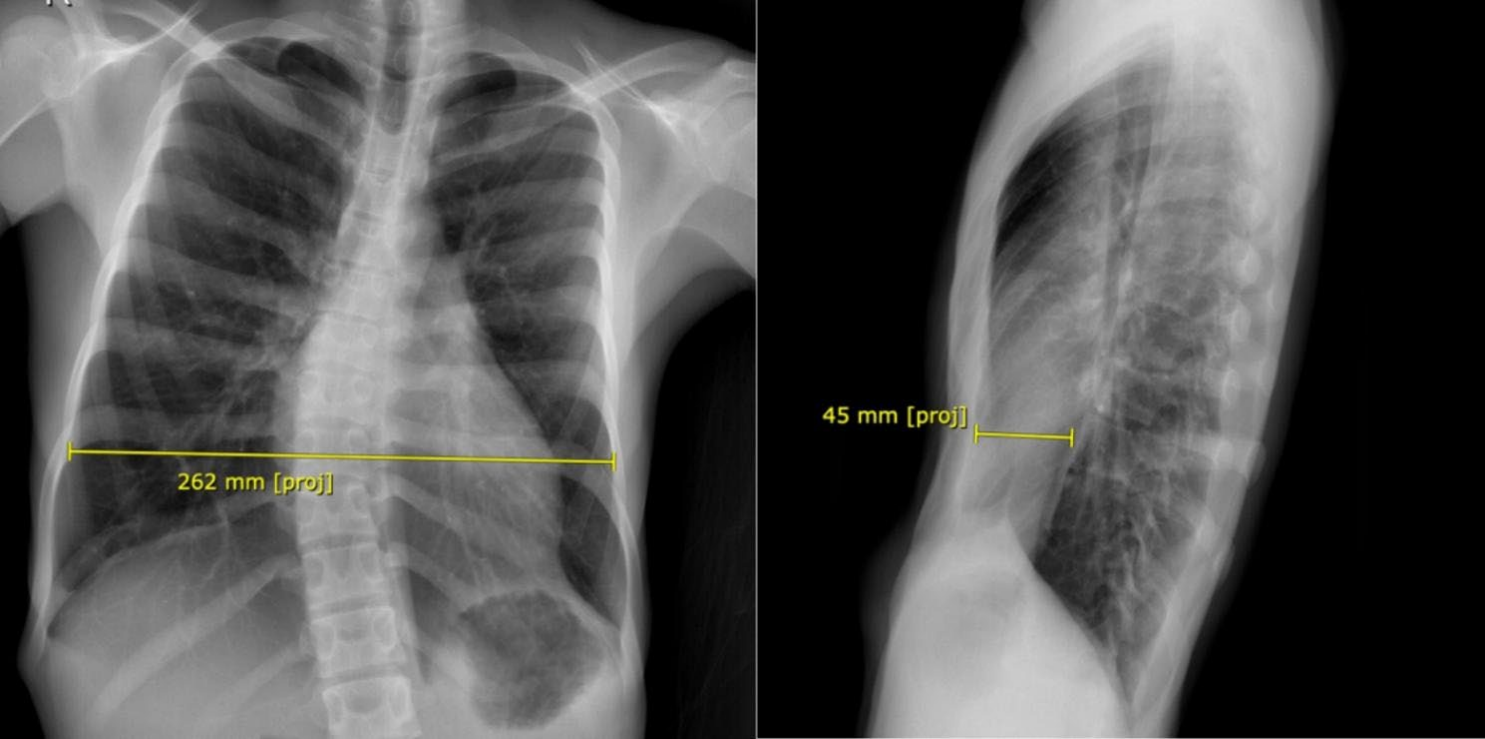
Preoperative chest X-ray. The transverse and anteroposterior diameters were measured with a Haller index of 5.8
